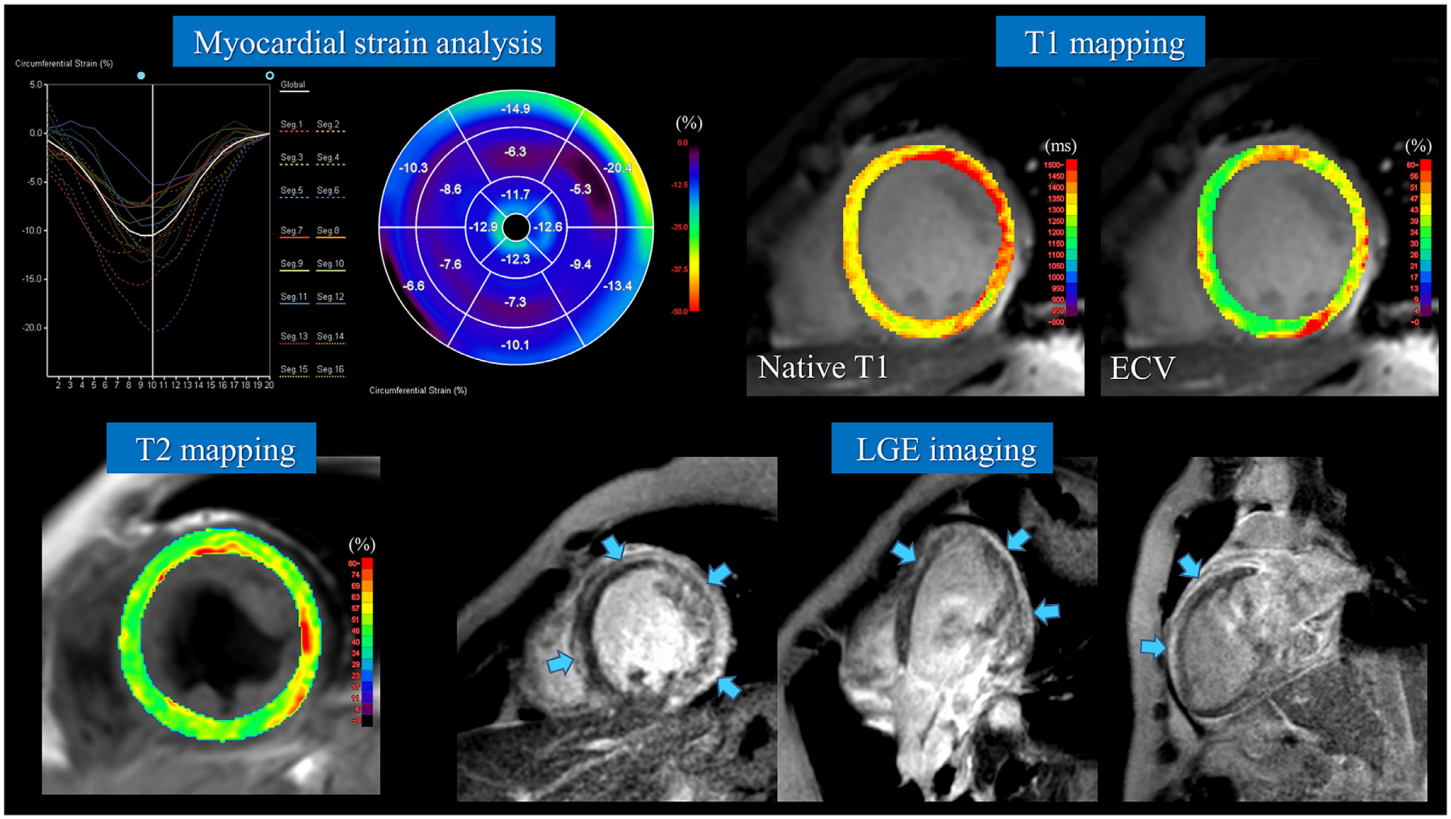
Multiparametric CMR of DMD-associated cardiomyopathy. Feature-tracking strain analysis showed reduced peak circumferential strain and marked temporal dispersion. T1 mapping demonstrated prolonged native T1 (1450 ms; reference: 1230-1250 ms) and elevated ECV (39%; reference: 23%-28%). T2 mapping revealed prolonged native T2 (53 ms; reference: 40-45 ms), suggestive of myocardial edema. LGE imaging showed a ring-like distribution of extensive subepicardial fibrosis in the LV myocardium. (MRI system: Ingenia CX, R5.4; Philips Healthcare, and image analysis workstation: Ziostation REVORAS; Ziosoft).
